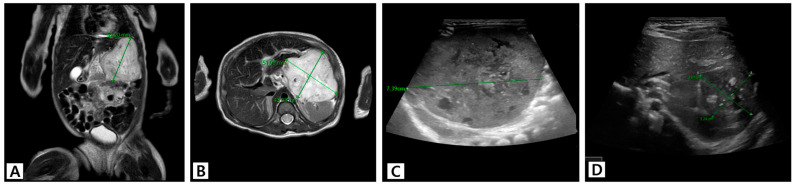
The US and MRI images of Patient 10, who had a large HH that partially resolved after step-by-step medical treatment. (A,B) MRI performed on the third day after birth shows an approximately 5.2 × 4.3 × 5.1 cm sized lobulated T2 hyperintense mass in the left lateral segment of the liver. The patient presented with feeding difficulty and abdominal distension. Oral propranolol was administered for 3 weeks from the 6th day of birth, and prednisolone was added at 1 month of age. (C) US performed at the age of 6 weeks revealed that the HH had increased in size up to 7.2 × 5.2 × 7.4 cm, with no improvement in symptoms. Oral sirolimus was initiated. (D) Follow-up US performed at 5 months of age shows that the tumor had decreased to 3.7 × 3.2 × 2.8 cm. Abbreviations: HH, hepatic hemangioma; US, ultrasound; MRI, magnetic resonance imaging.
